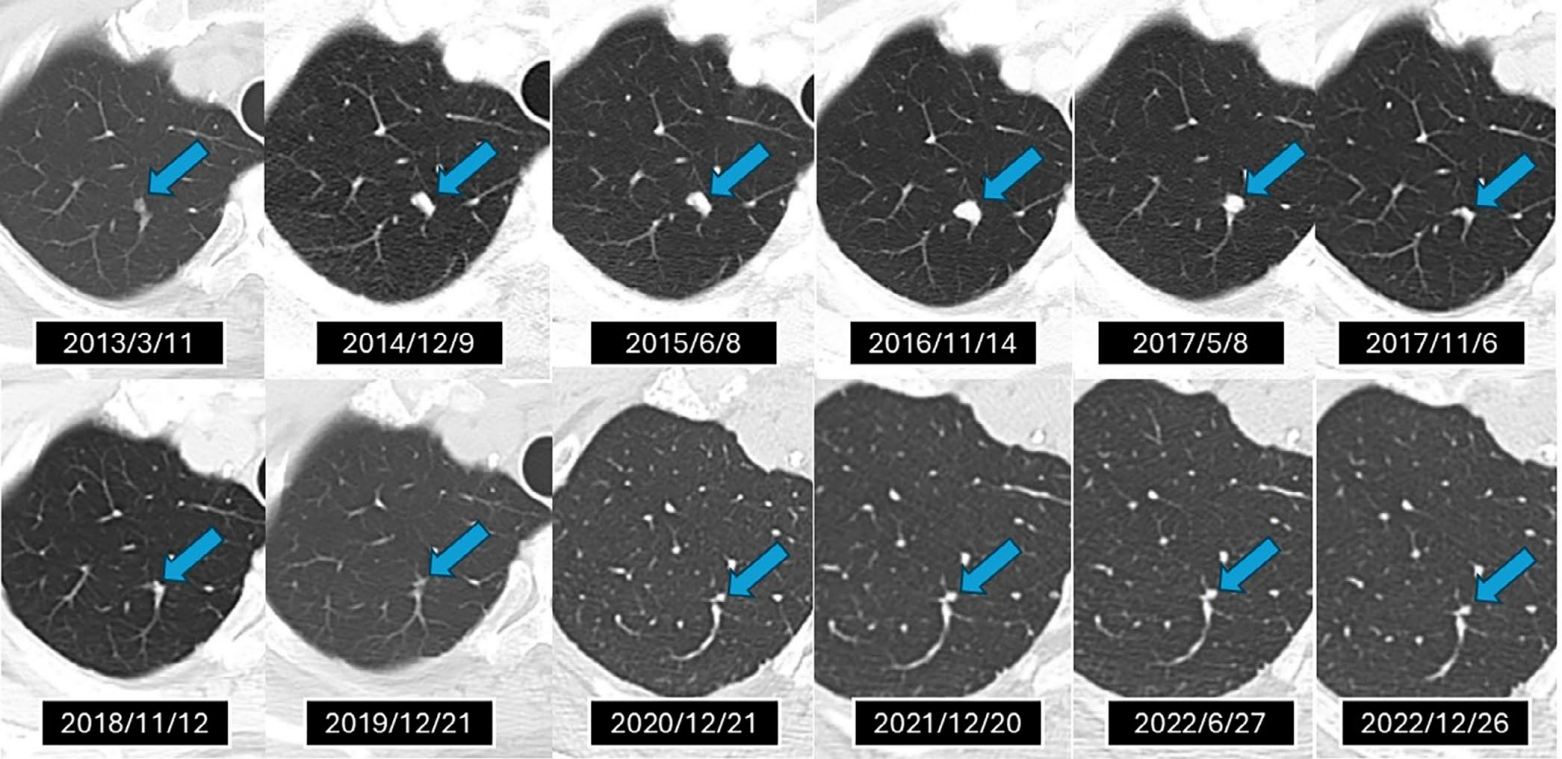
Radiographic course of the pulmonary metastasis demonstrating an abscopal response following stereotactic body radiation therapy (SBRT) with the CyberKnife system. A small ground‐glass nodule in the right lung was retrospectively identified on a CT scan from March 2013, prior to SBRT to the left renal lesion in April 2013. The nodule gradually enlarged, peaking in size by November 2016, then spontaneously regressed without further treatment and remained stably reduced in size thereafter. Serial imaging through December 2022 confirmed its sustained size reduction, with no CT scans performed thereafter.
